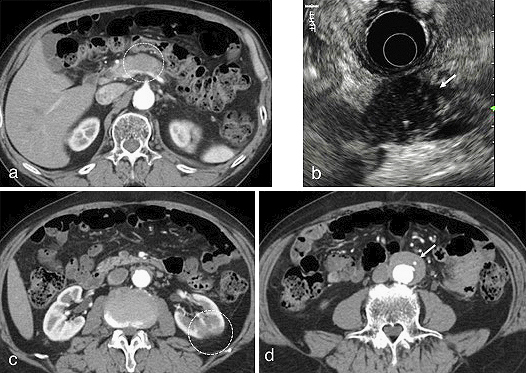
Image findings of a 72-year-old man with focal AIP. (a) Contrast-enhanced CT disclosed a localized attenuated mass at the pancreatic body (circle). (b) EUS showed a low-echo pancreatic mass with high-echo spots (arrow). (c) Contrast-enhanced CT revealed left kidney involvement with renal cortical lesions of decreased enhancement (circle). (d) Contrast-enhanced CT showed a soft tissue mass around the abdominal aorta (arrow).
